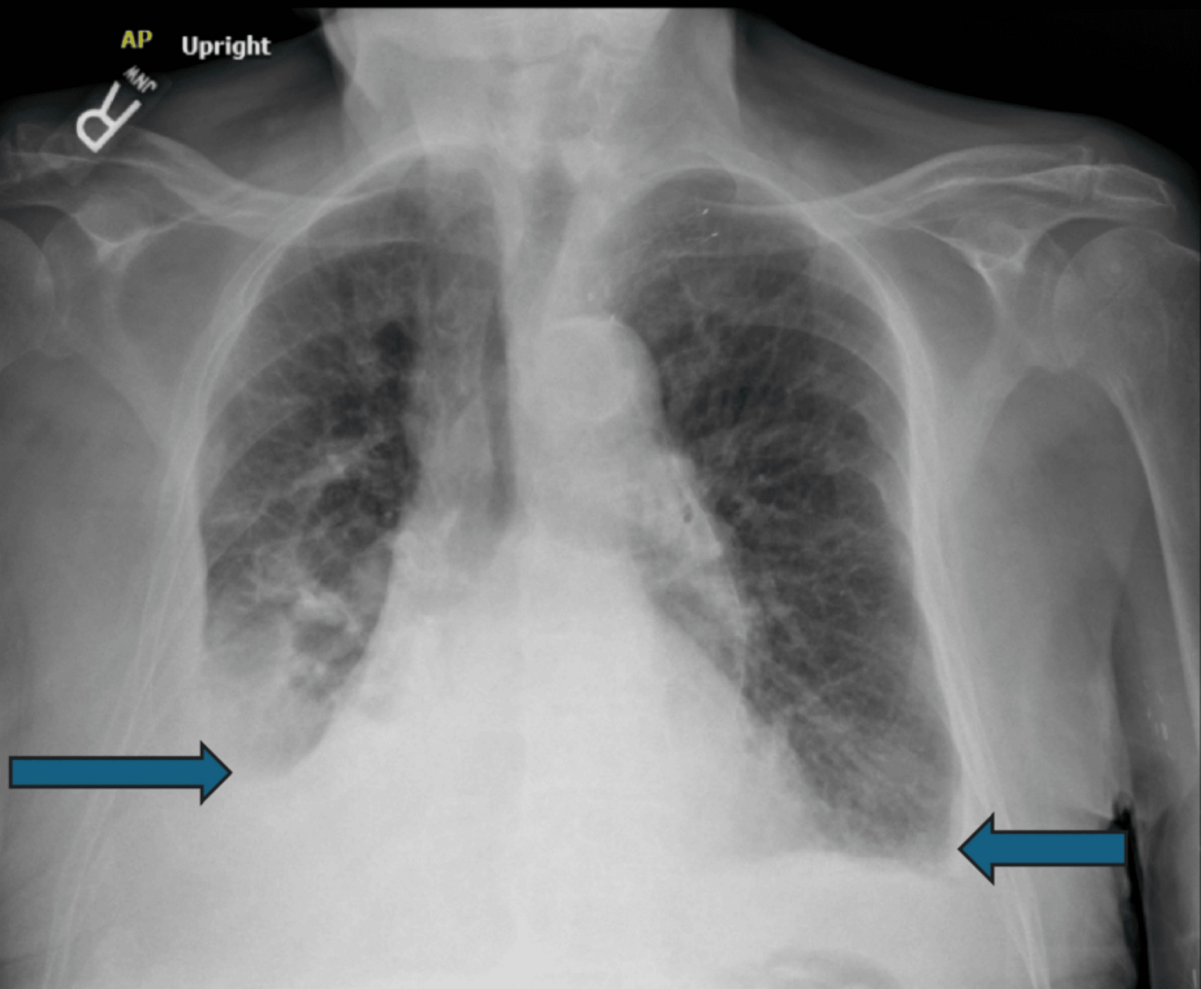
Chest radiograph in May 2025, one day before the ultrasound-guided bilateral thoracentesis
